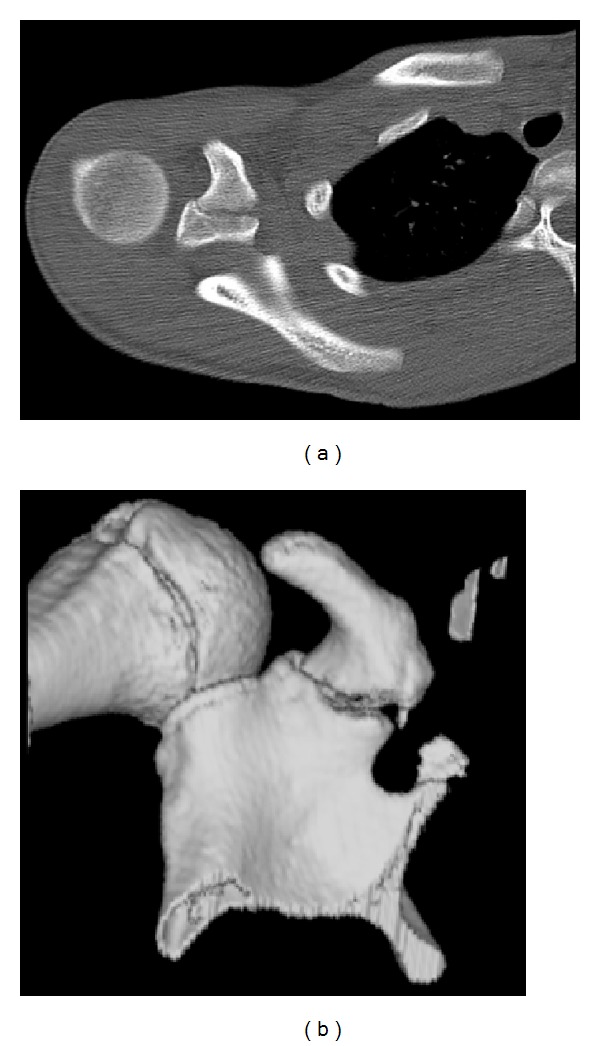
Case A: axial CT scan and CT 3D reconstruction.
